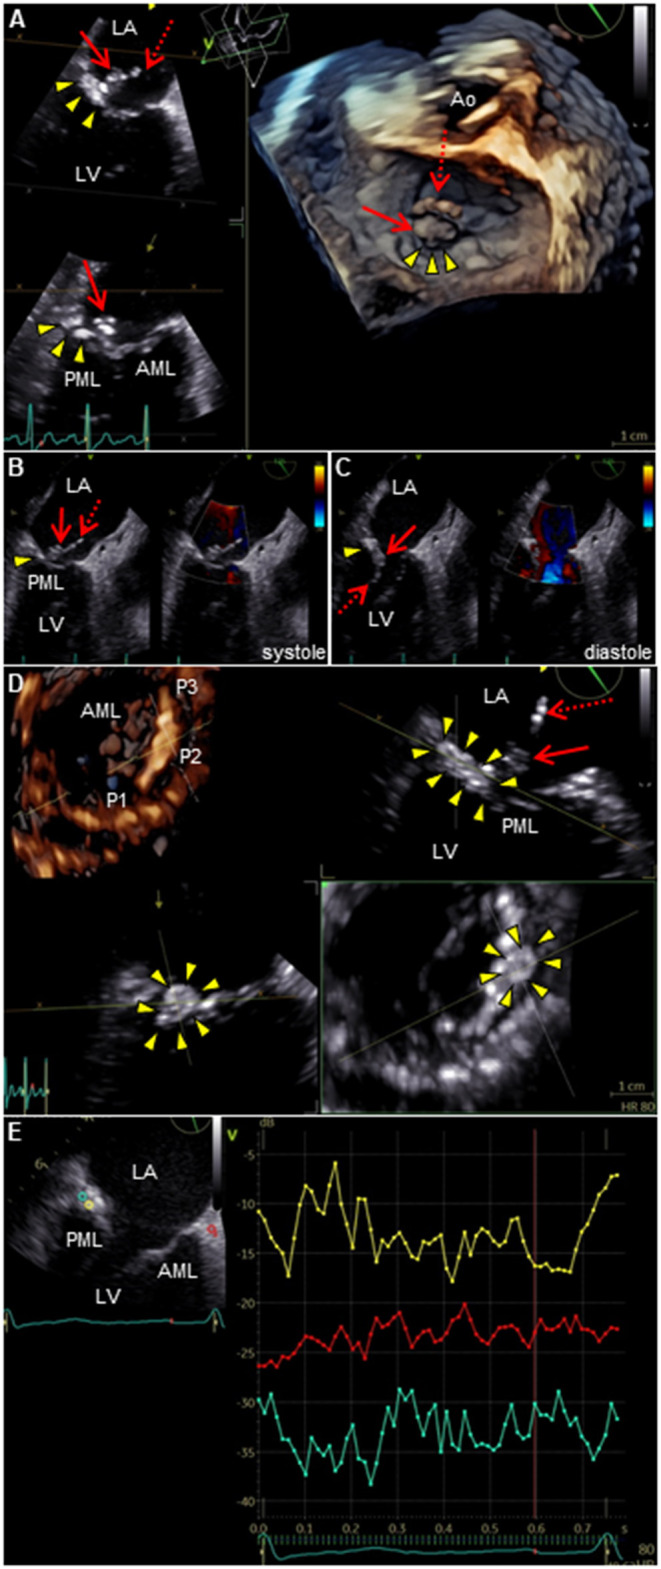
TEE of the mitral valve. (A) 3D TEE or (B,C) 2D color Doppler TEE shows a long mobile mass of vegetations which consist of body part (arrow) and tail part (dotted arrow), containing calcium-dense stippling, attached to the calcified posterior mitral annulus (arrowheads). Note the intact valve leaflets. (D) 4D TEE with Flexi-slice mode demonstrates MAC with a central echolucent area (arrowheads) around the P2 segment of the mitral valve. (E) Q-analysis presents a contrast analysis that traces the grayscale intensity in a defined region of interest as a function of time: green, yellow, and red depicts the center of MAC, the periphery of MAC, and normal cardiac muscle tissue as a control, respectively (left). Time-intensity curves are plotted for the respective region of interest (right): X axis, Time (s); and Y axis, Intensity scale (logarithmic) (dB). AML, anterior mitral leaflet; Ao, aorta; LA, left atrium; LV, left ventricle; MAC, mitral annular calcification; PML, posterior mitral leaflet; TEE, transesophageal echocardiography.
